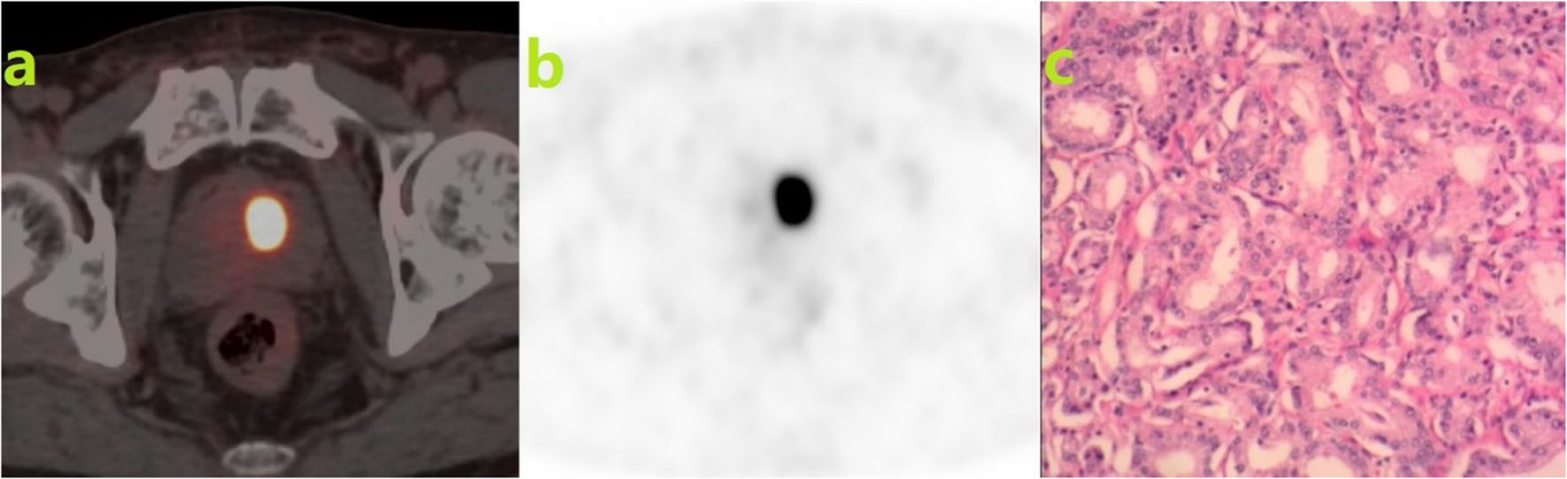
A 79-year-old male patient. Physical examination revealed elevated blood PSA (8.85 ng/ml). He did not usually have difficulty urinating. A nodular focus of higher radioactive uptake was observed in the left transition zone (a), with an SUV_max_ of 29.18 (b). Prostate cancer was confirmed by pathology: Gleason score: 3 + 4 = 7 (c)
